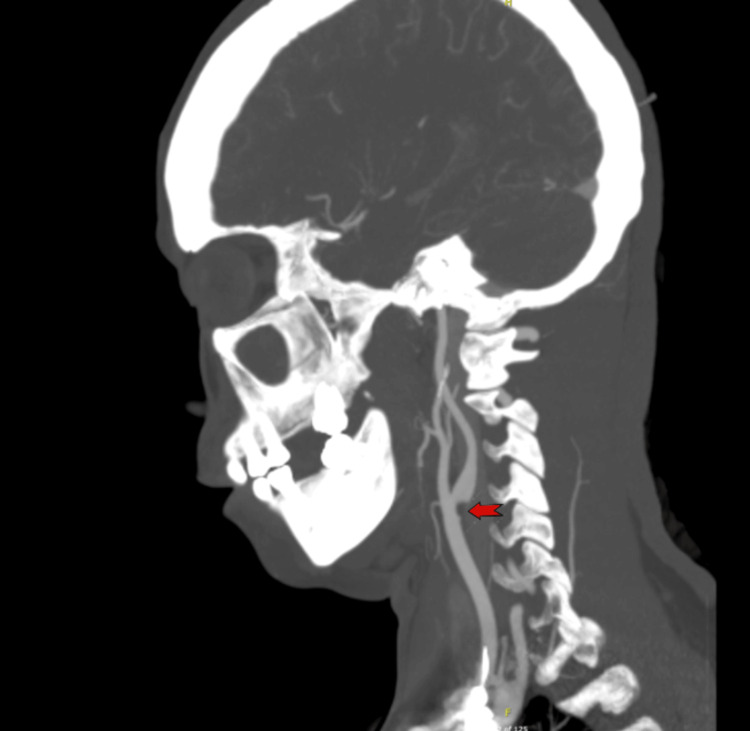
CT angiography (Sagittal view) It revealed hypodensities in the right frontal and temporal regions and occlusion of the superior and inferior branches of the M3 segment of the right middle cerebral artery, and a focal filling defect at the carotid bulb/origin of the right internal carotid artery as shown by the red arrow, likely due to thrombus formation from dissection.
